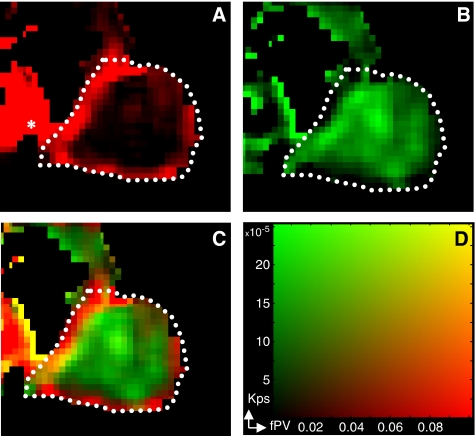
MRI mixed images. An fPV map in red (A) and Kps map in green (B) were mixed to produce the image (C) by the corresponding colour scale (D). Yellow pixels in the mixed images indicate high fPV and high Kps. In (A) white dotted lines represent a manually drawn region encompassing the whole tumour. The central section of the tumour is shown, and the heart is visible left of the tumour (*).
